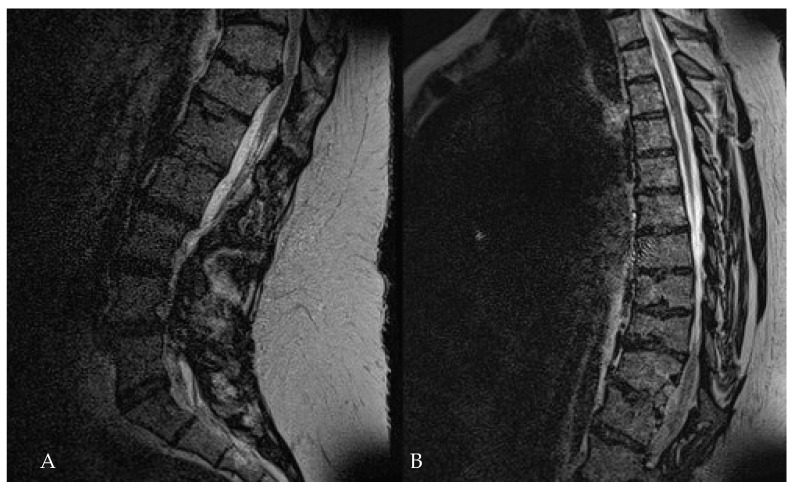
Patient No. 6. A. Preoperative T2-weighted sagittal MRI of the lumbar spine shows the herniated disc at Th11–12 level, with (B) the postoperative sagittal T2-weighted MRI of the thoracic spine, which shows the complete resection of the disc.
